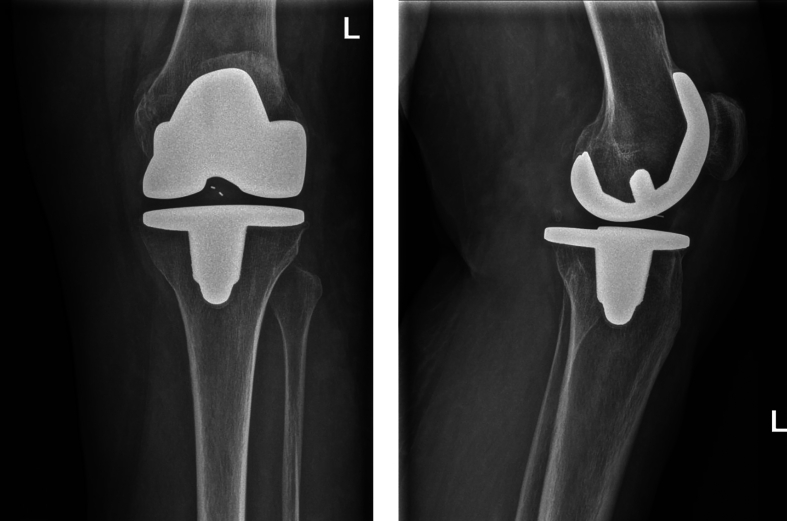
Case 1: conventional radiography images during follow-up.
